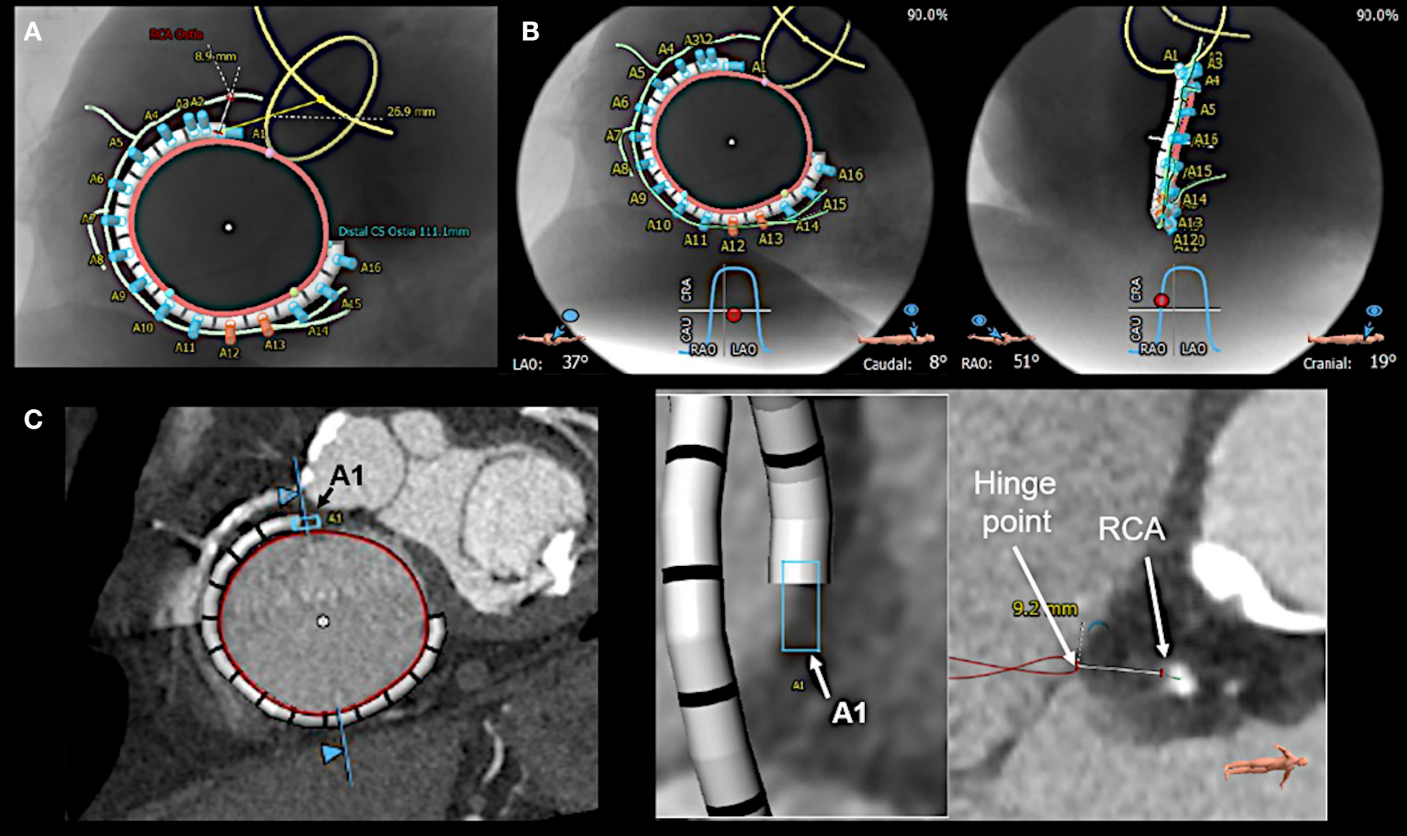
Multi-detector computed tomography (MDCT) based pre-procedural planning by using specific software (3mensio, Pie Medical Imaging BV, Bilthoven, the Netherlands). (A) MDCT is used to provide measurements of tricuspid annular (red circle) dimension and the distances of the anticipated anchor positions to the right coronary artery (RCA; light green line). Anchors with insufficient distance to the RCA are shown in red, the others in blue. Furthermore, the band size is determined, and the position of the first anchor point is defined as the distance from the antero-septal tricuspid annulus to the center of the aortic valve (yellow circle), which serves as a largely stable landmark. (B) In addition, optimal left anterior oblique (LAO; left) and right anterior oblique (RAO; right) projections for the procedure are pre-defined. (C) For each anticipated anchor position, the distance to the RCA and the hinge point of the leaflet is measured. Here the first anchor (A1) position is exemplarily shown.
